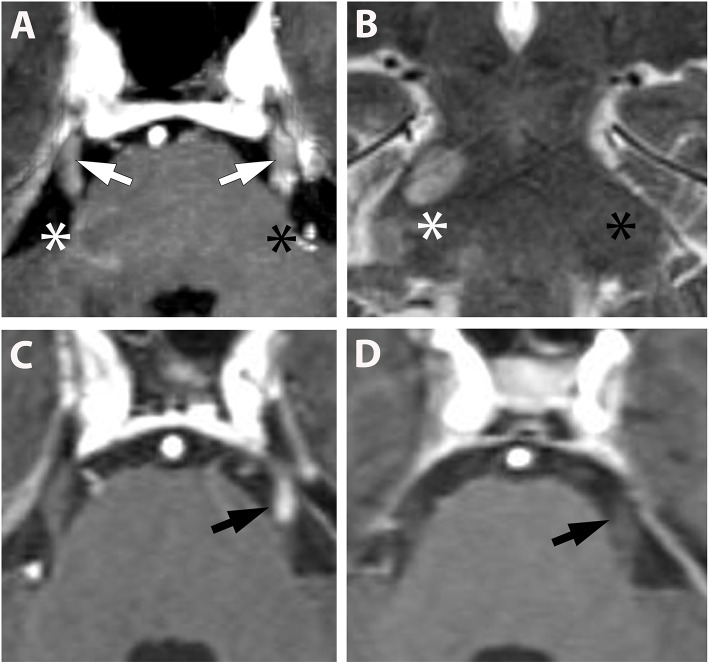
(A,B) (Pat. No. 2): Axial T1-weighted MRI post contrast enhancement (A) at the level of the pons and a corresponding coronal T2-weighted MRI of the same patient (B). On post contrast images, intense bilateral contrast enhancement is depicted in the cisternal segment of the trigeminal nerve (white arrows). A ring-enhancing lesion is located at the root entry zone of the right trigeminal nerve (white asterisk) (A). No root entry zone lesion is visualized at the contralateral site (black asterisk) (A,B). (C,D) (Pat. No. 11): Axial T1-weighted MRIs post contrast enhancement (A,B) at the level of the pons, at baseline (C), and at follow-up MRI 11 months later (D).
